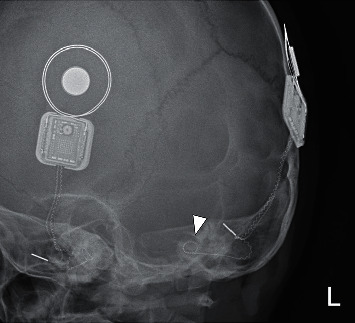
Lateral skull X-ray after initial cochlear implantation. The arrowhead points to the electrode which is curled in a 360-degree position, indicating its position in the basal and middle turn.
